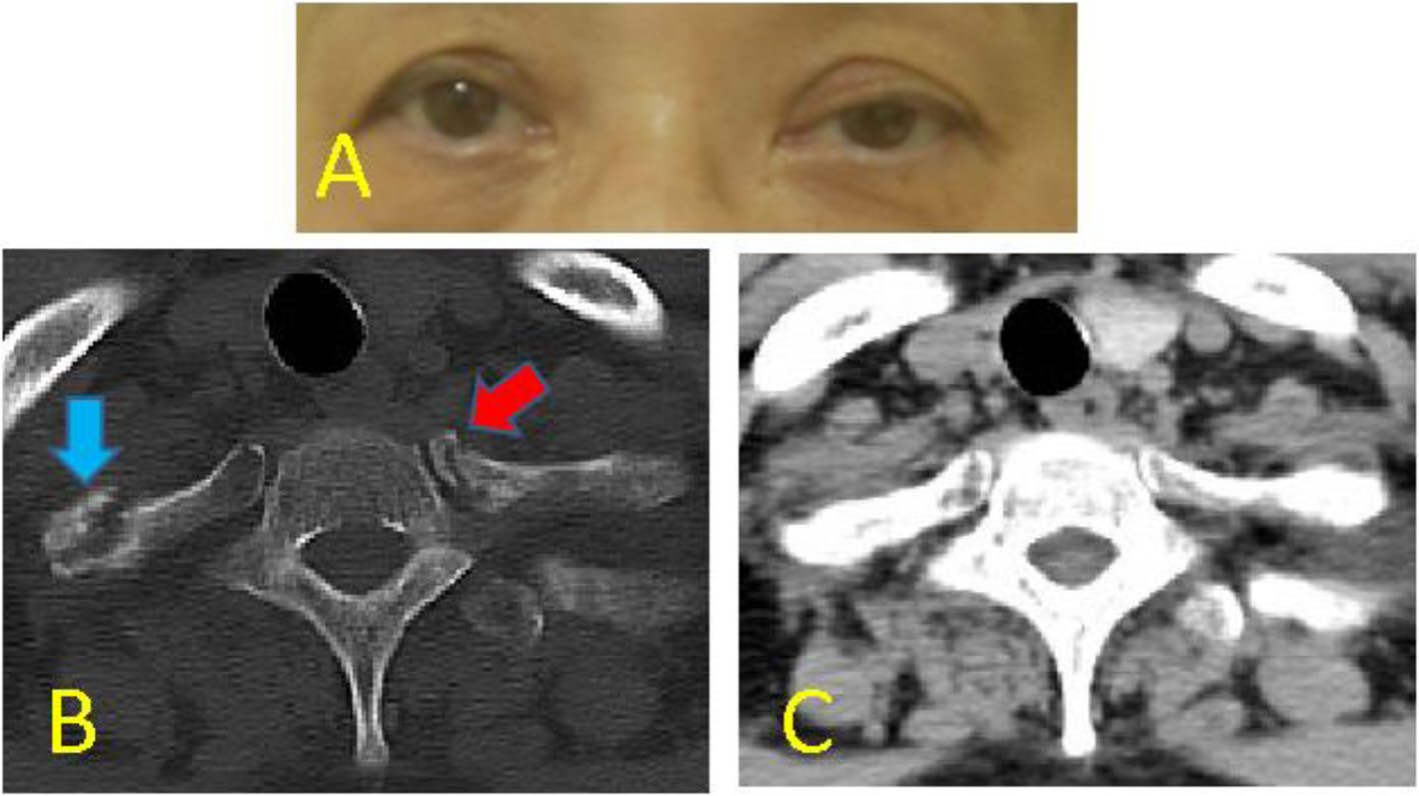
Findings before and after CABG. A Left ptosis appeared after discharge and she was diagnosed with Horner’s syndrome on postoperative day (POD) 48. B Plain CT scan on POD 76 revealed displaced fractures of the right first rib neck (blue arrow) and the left first rib head (red arrow). C Plain CT scan before CABG. Compared to this image, the bone fragment of the left first rib head (B, red arrow) was shown to be displaced 3 mm anteriorly, which suggested that the fragment affected the stellate ganglion in the sympathetic trunk
